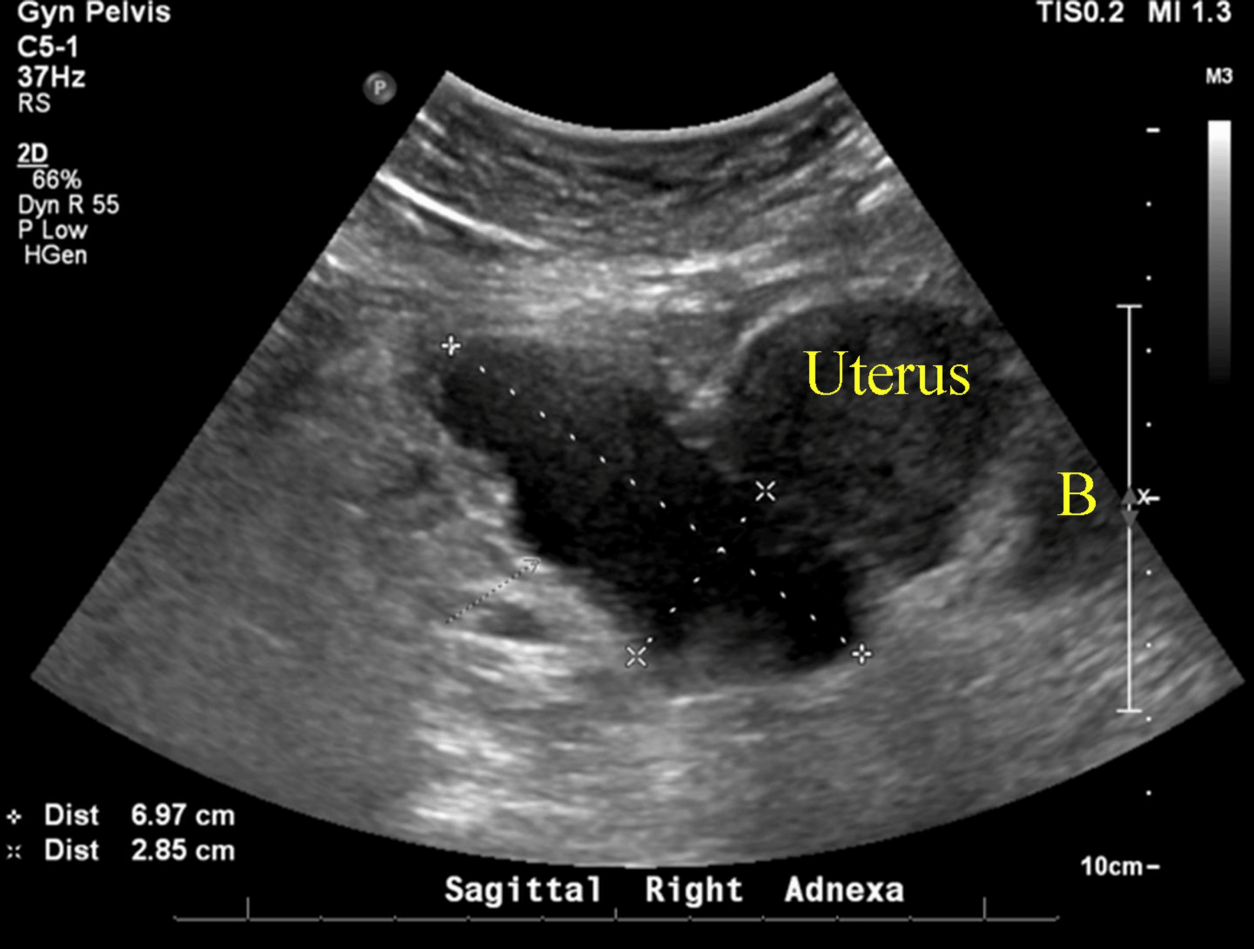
Transvaginal ultrasound demonstrating a tubo-ovarian abscess (TOA) measuring 6.9 x 2.8 cm. B: bladder.
